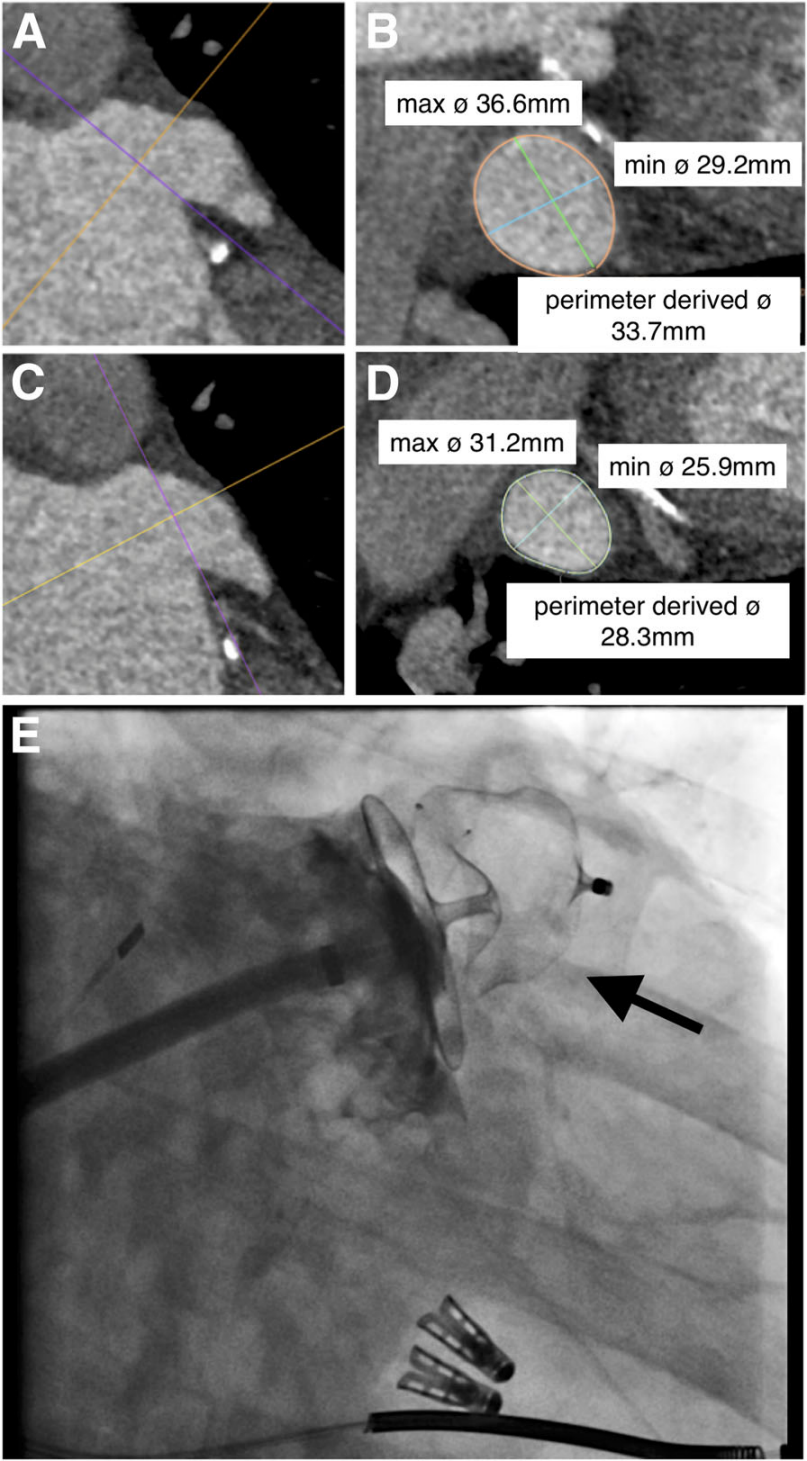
a-b CT measurements of the usual landing zone, and c-d CT measurements of a more distal alternative landing zone. e Amulet LA closure device released with signs of adequate stability and minimal leakage. CT, computed tomography
